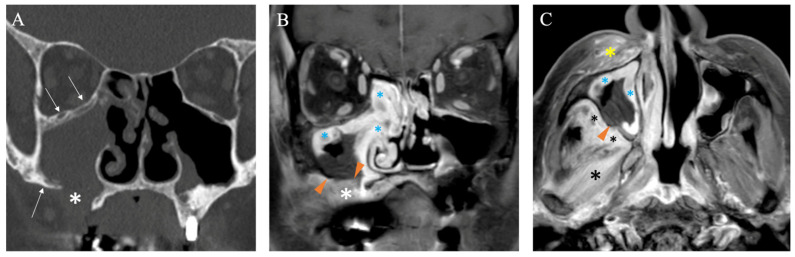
71-year-old female confirmed with right AIFR (*Mucor*). Coronal CT (A) shows non-specific opacification of the right maxillary sinus. The bony sinus walls are thickened (arrows), and there is an anterior-inferior defect (white asterisk) caused by a Caldwell-Luc procedure performed in the past. Coronal (B) and axial (C) contrast-enhanced T1W fat-saturated MR images reveal inflamed, strongly enhancing mucosa (blue asterisks), along with areas of non-enhancing mucosa (arrowheads) that strongly suggest AIFR. There is infectious involvement of the retro-antral fat (small black asterisks), pterygoid muscles (large black asterisk), and subcutaneous cheek fat (yellow asterisk). See Figure 1C.
